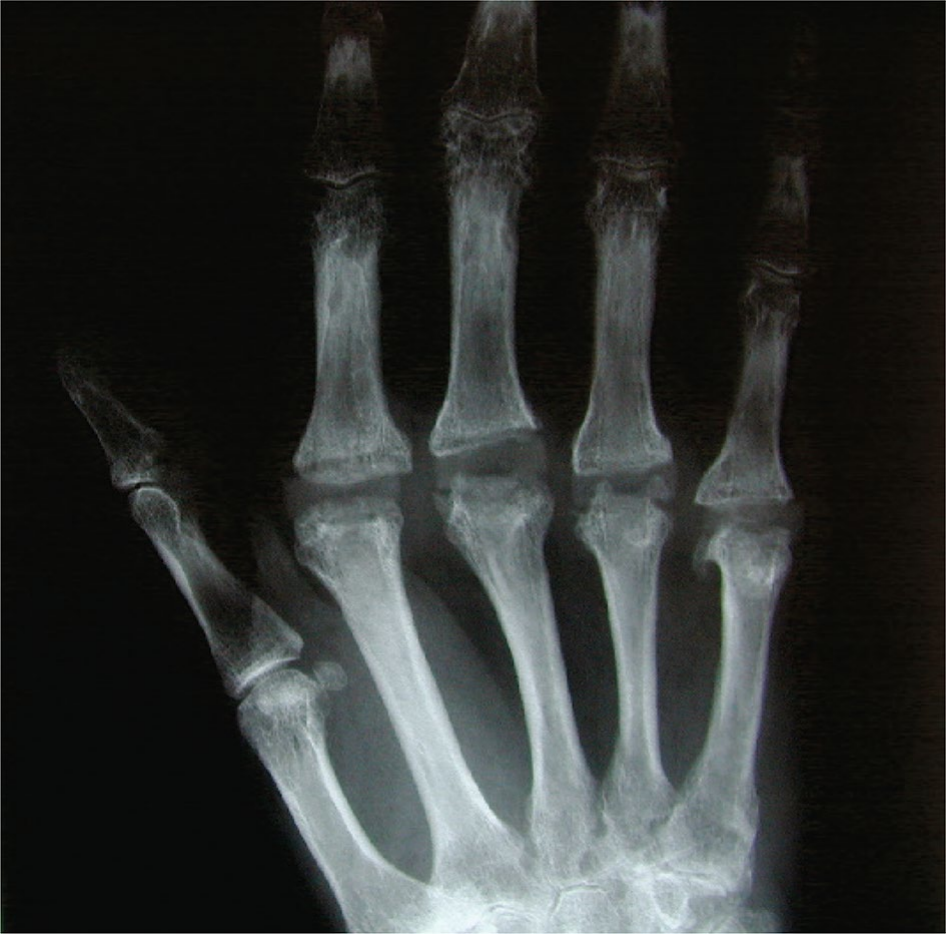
The postoperative radiographic appearance of the Swanson silastic MCPJ arthroplasty. Radiographs show no evidence for implant failure
